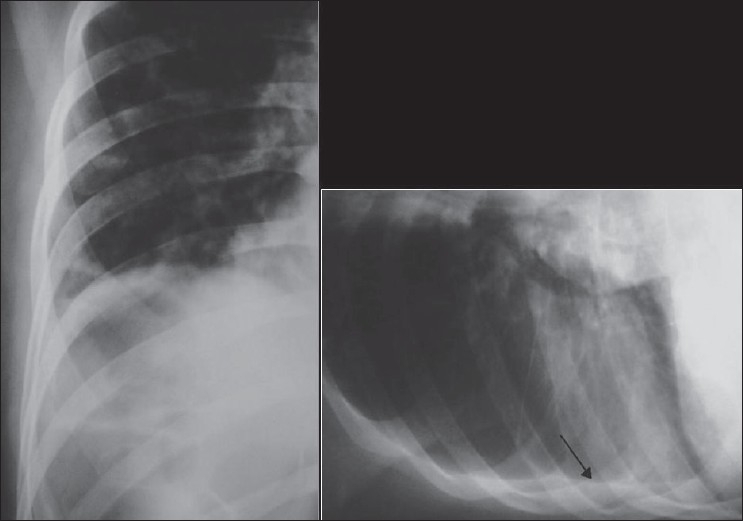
Radiographs showing the value of lateral radiography in the detection of smaller pleural effusions. There is blunting of the right costophrenic angle on the erect radiograph (left), but the appearances are not diagnostic of a pleural effusion. However, the lateral decubitus film (right) shows layering of the pleural fluid (arrow)
